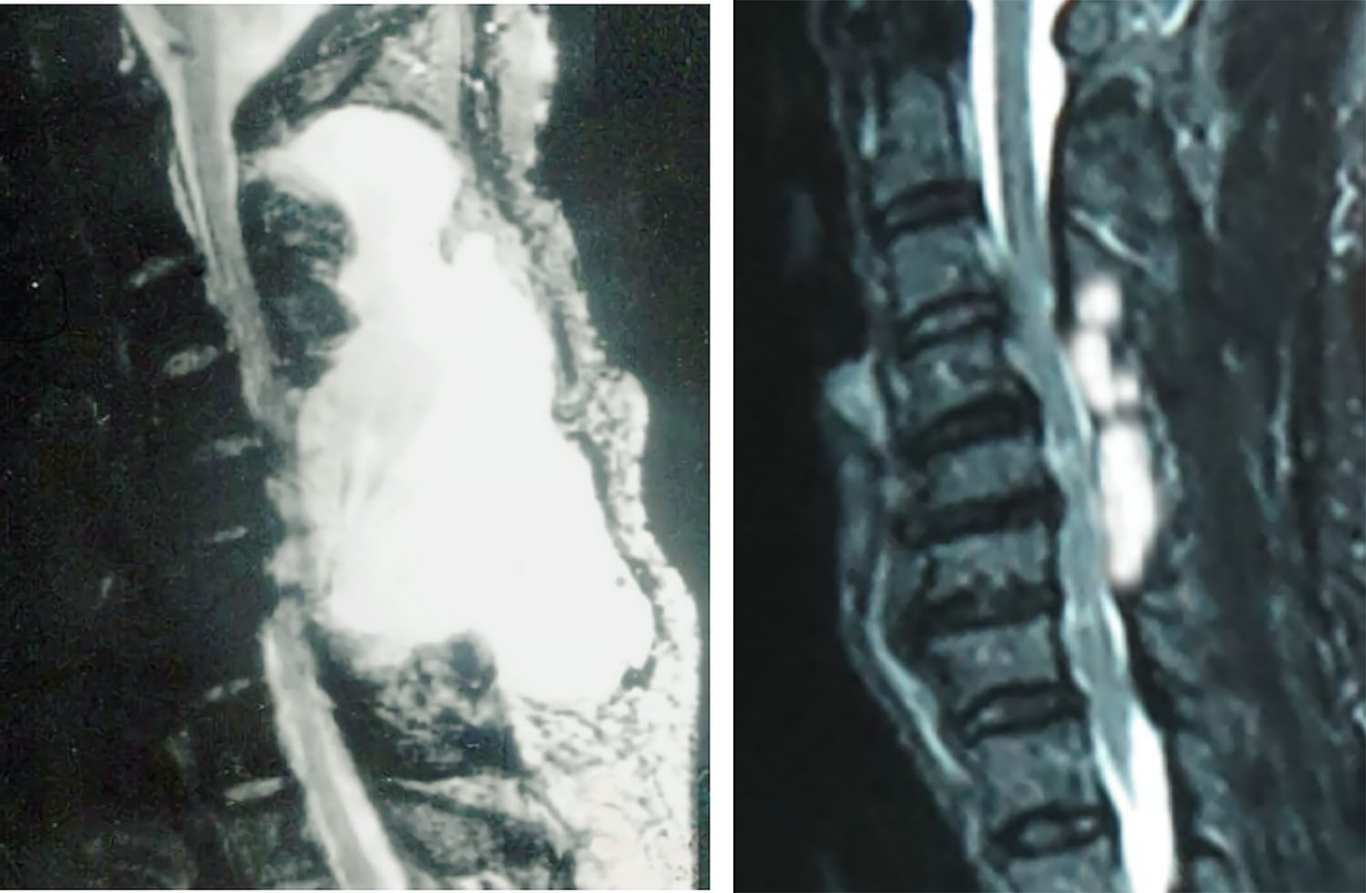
The second case. (a) Pre-operative area of the pseudomeningocele on sagittal MRI section. (b) Post-operative area of the pseudomeningocele on sagittal MRI section.
